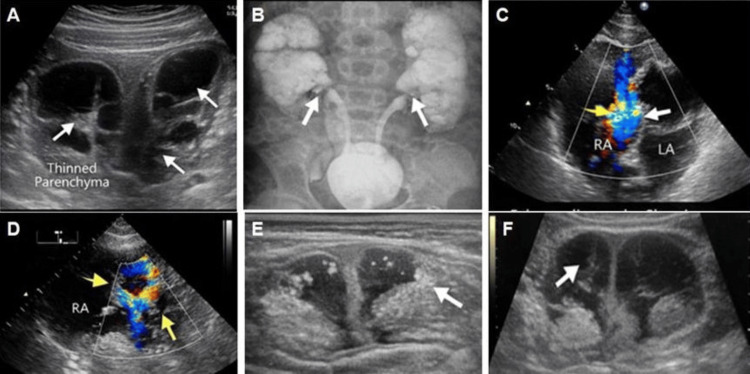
Imaging studies A) Bilateral grade IV hydronephrosis with parenchymal thinning; B) VCG/MCUG showing bilateral vesicoureteral Reflux; C, D) Echocardiography showing large secundum ASD with moderate right heart dilatation; D) Echocardiography showing large secundum ASD with moderate right heart dilatation; E, F) USG showing lumbar soft tissue lesion.
